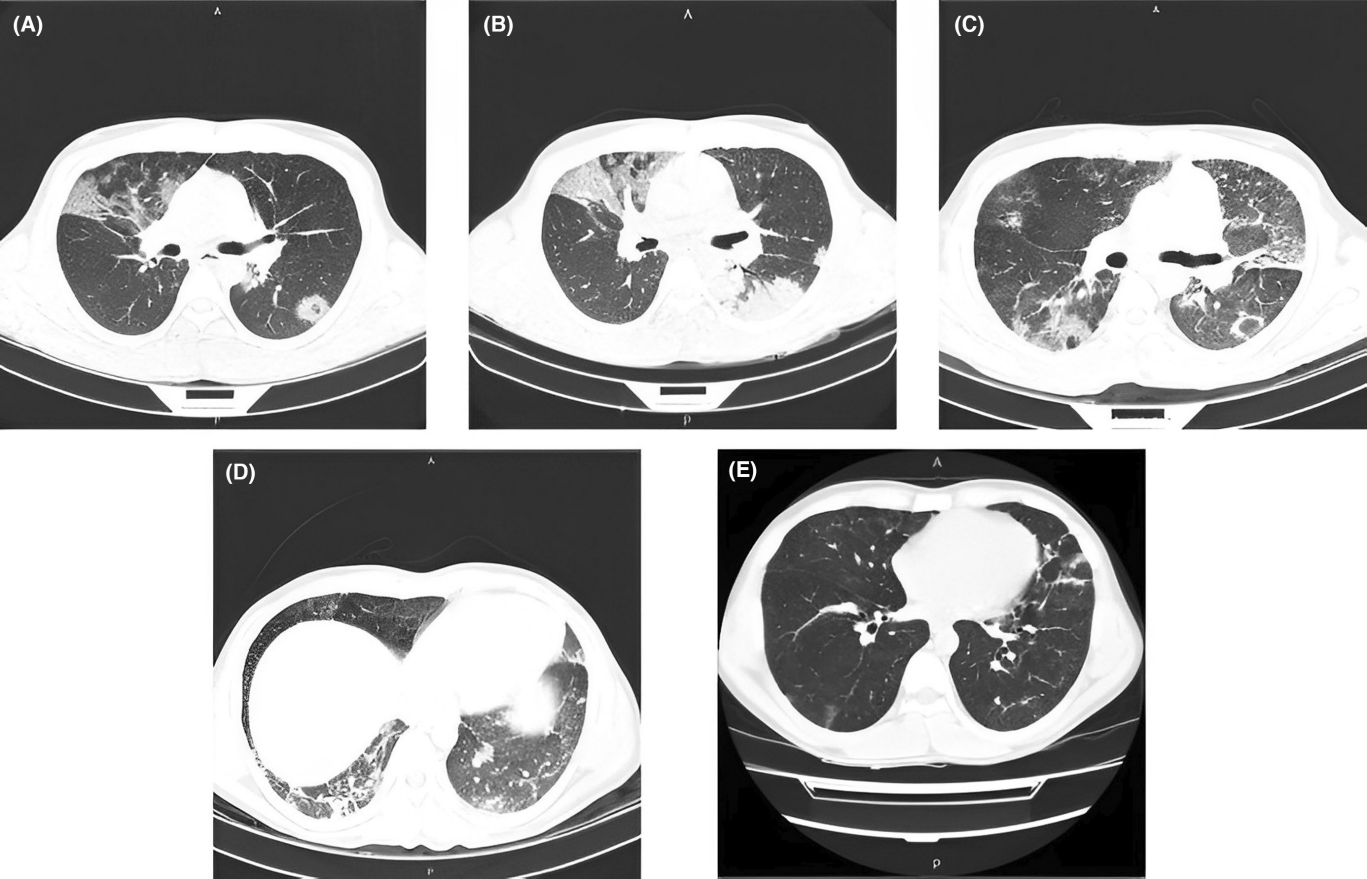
(A) on admission day CT scan, there were multilobal bilateral patchy consolidations, ground glass opacities, and interlobular septal thickening, with the peripheral and peri‐bronchovascular distribution. “Crazy paving” is the dominant feature at the right upper lobe; (B) CT scan right before bronchoscopy showed increased consolidations, ground glass opacities, and crazy paving in the same manner at multiple lung segments; (C, D) on discharge day CT scan, there were decreased consolidations and change to ground glass opacities and crazy paving appearance at the same involved segments. There was also evidence of “reverse halo” in several segments and progression to fibrotic changes predominantly at lung bases, as parenchymal and subpleural bands; (E) CT scan image, 3 months after discharge.
